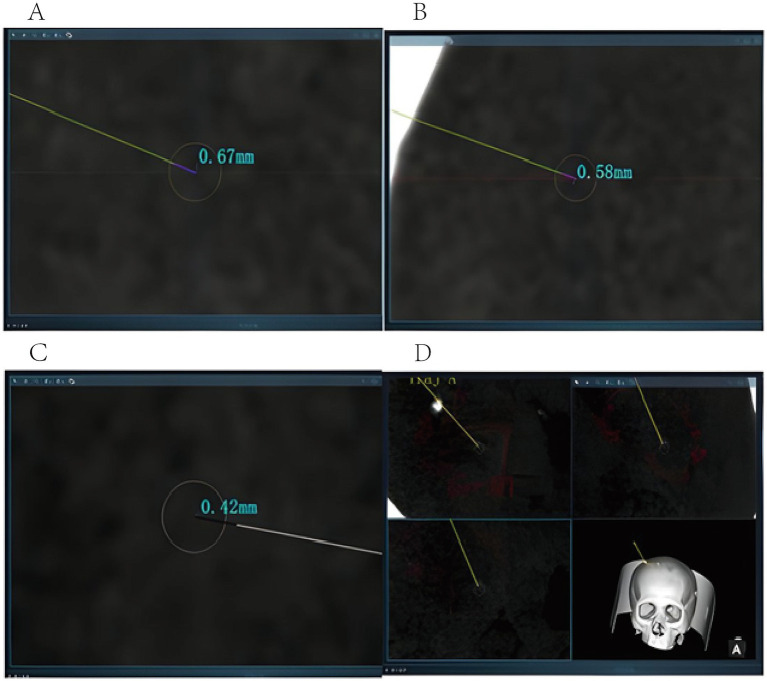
Target error calculation: (A) coronal operative target error 0.67 mm; (B) sagittal operative target error 0.58 mm; (C) axial operative target error 0.42 mm; the mean error was 0.55 mm; (D) fusion of the planned surgical trajectory with postoperative CT.
